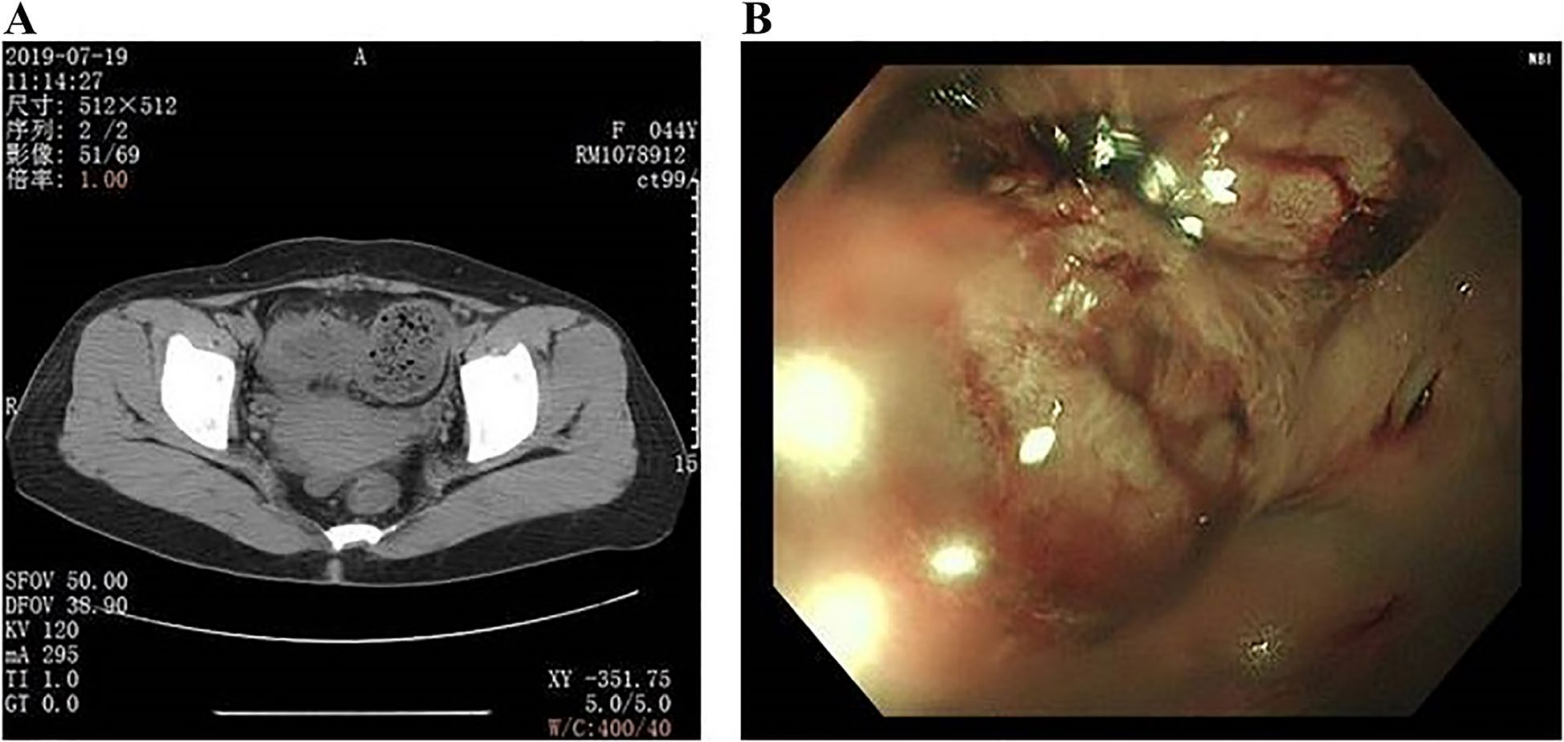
A Abdominal computed tomography (CT) showed thickening of intestinal wall at the junction of sigmoid colon and descending colon. B Colonoscopy revealed a colonic mass
